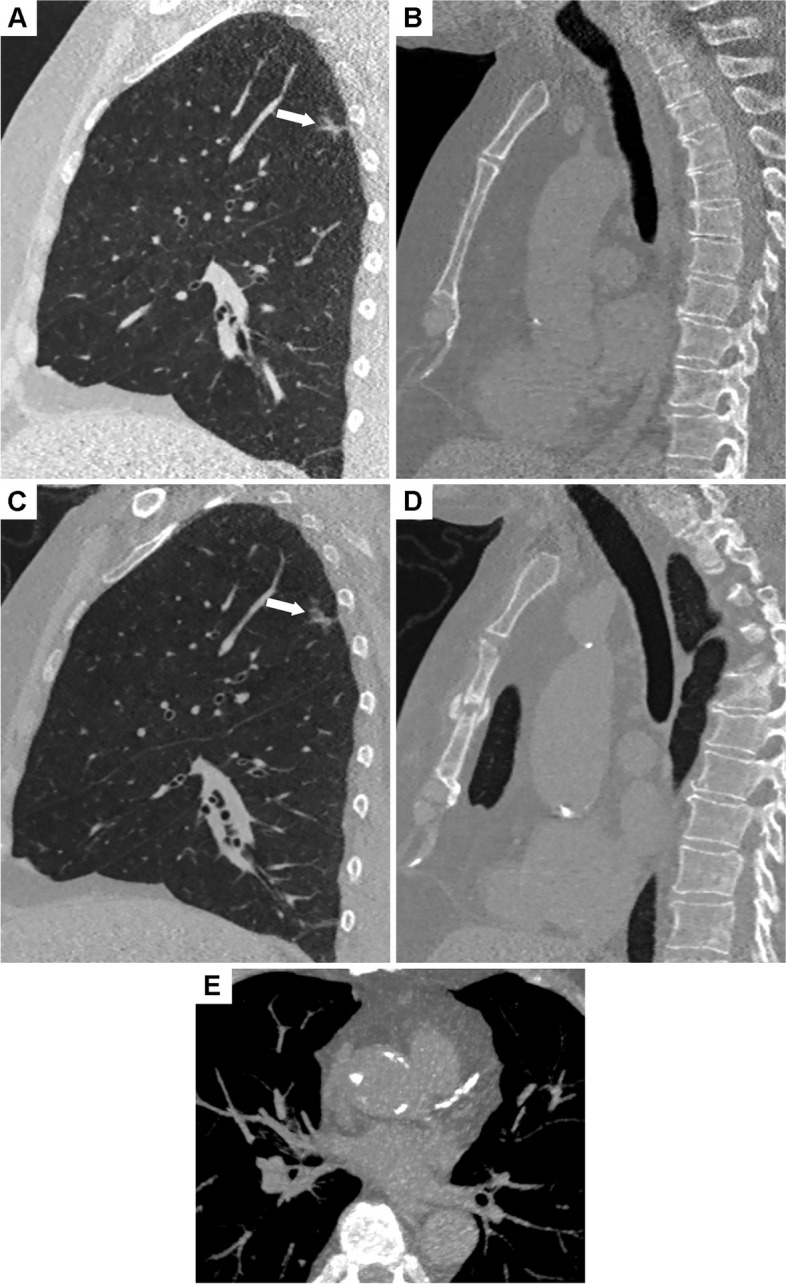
A sagittal reformat of a baseline CT scan in a 61-year-old participant shows an irregular subsolid nodule in the right upper lobe (arrow) (A), with the sternum showing no abnormalities (B). CT follow-up at 3 months (C) showed no change in the morphologically suspicious nodule (arrow), but a recent fracture of the sternum (D). This was due to cardiac massage for a cardiac arrest secondary to a myocardial infarction which occurred in the interval between the two CT scans. On the baseline CT, the left descending coronary artery was heavily calcified (E). A segmentectomy was performed after recovery, revealing an invasive acinar adenocarcinoma staged pT1a No Mo
